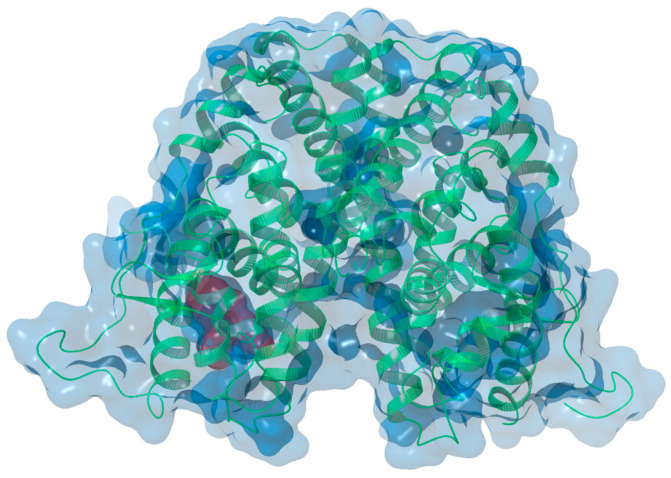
X-ray structure of the ER ligand binding pocket (PDB code 7KBS [40]).
